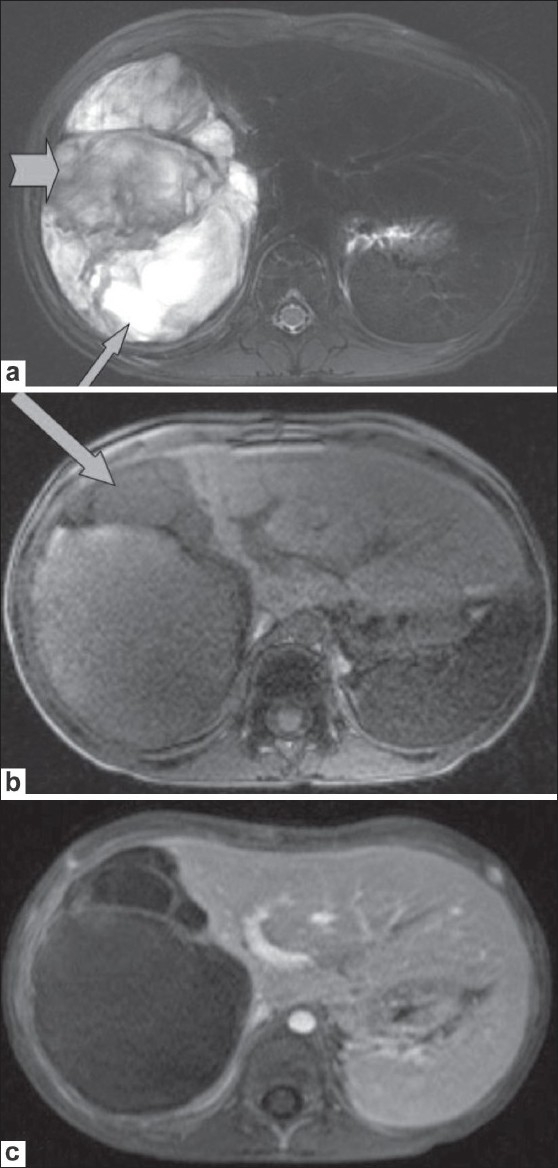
T2W axial MRI of the abdomen (a) shows the mass to be predominantly hyperintense (arrows), with hypointense areas (arrowheads) within. A T1W axial MRI image (b) shows the lesion to be iso to heterogeneously hypointense (arrows). A contrast-enhanced T1W axial MRI image (c) shows absence of enhancement
